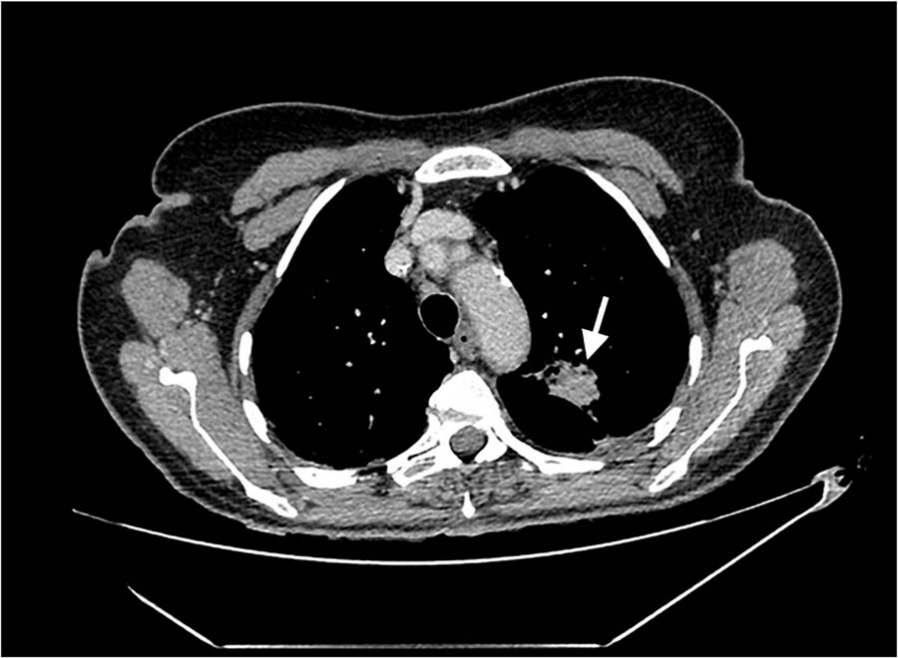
Axial CT image of the lungs, showing a cavitating mass in the posterior aspect of the left upper lobe, suggestive of an abscess secondary to infective endocarditis.
